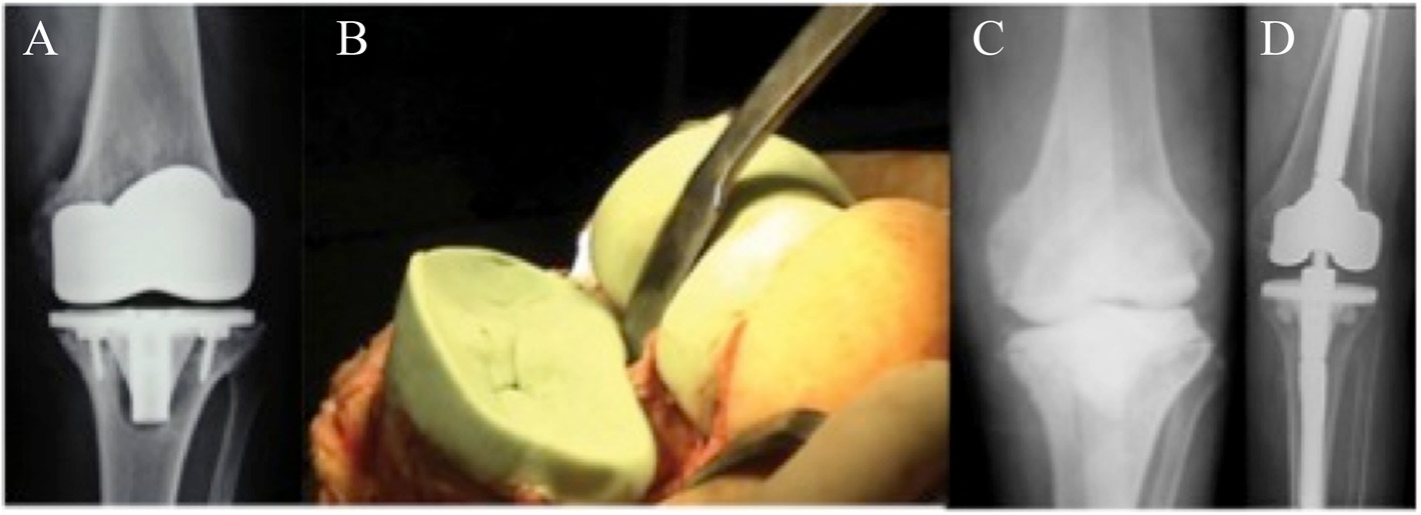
74 y, male: 4 years after TKA with septic loosening and medial tibial collapse A) preoperative x-ray B) Two stage revision with hand made antibiotic-loaded articulating cement spacer. C) Postoperative x-ray a.p. view D) Second stage revision after an interval of 8 weeks with condylar constraint prosthesis.
